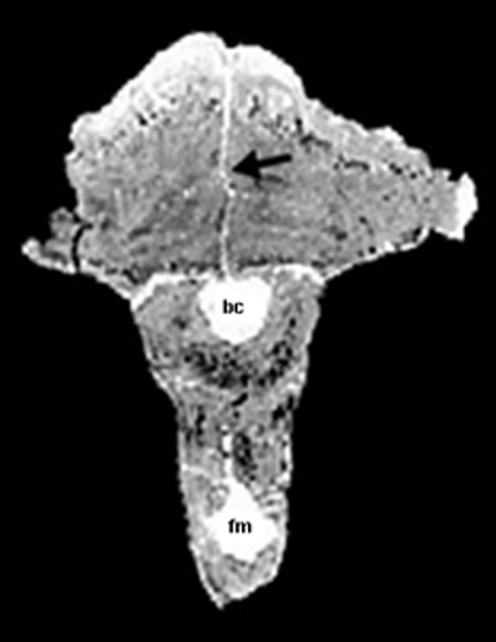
A coronal CT scan through the dome of “*Stygimoloch*” (MPM 8111). The intrafrontal suture (black arrow) is open internally supporting the subadult status of this pachycephalosaurid. The braincase (bc) and foramen magnum (fm) are clearly visible.
